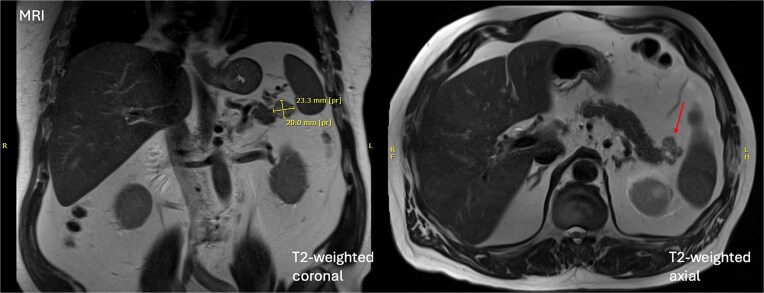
Initial MRI demonstrating a 20 × 26 × 20 mm mixed solid and cystic lesion in the pancreatic tail.
